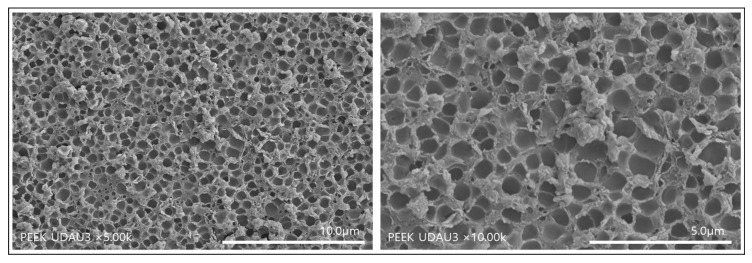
SEM images of PEEK implants at various magnifications after treatment with a mixed acid of sulfuric acid and nitric acid under ultrasound for 90 s and then washing with distilled water for 6 h under ultrasound.
